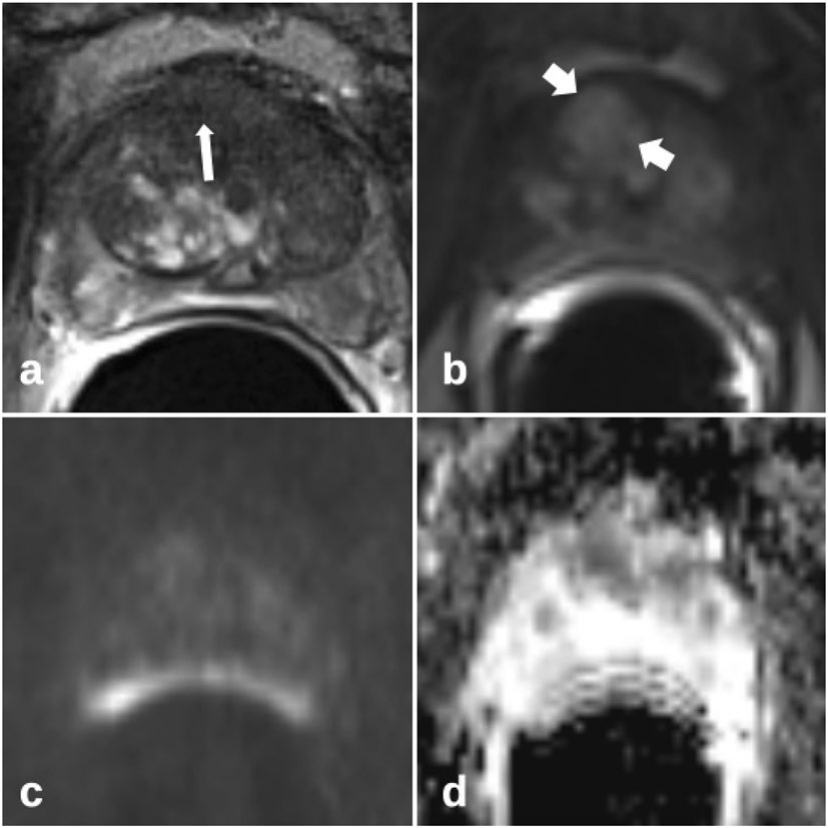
Homogenous perfusion pattern of transition zone prostate carcinoma (short arrows in b) with additional asymmetry improves lesion detection compared to T2-weighted and diffusion-weighted imaging alone; patient of 70 years, PSA 29 ng/ml, prostate volume 41 ml, prostate carcinoma G3 Gleason 4 + 4; a T2-weighted axial imaging with marked biopsy trace (long arrow); b T1w early-phase dynamic perfusion; c diffusion-weighted imaging with b = 1400; d apparent diffusion coefficient map
